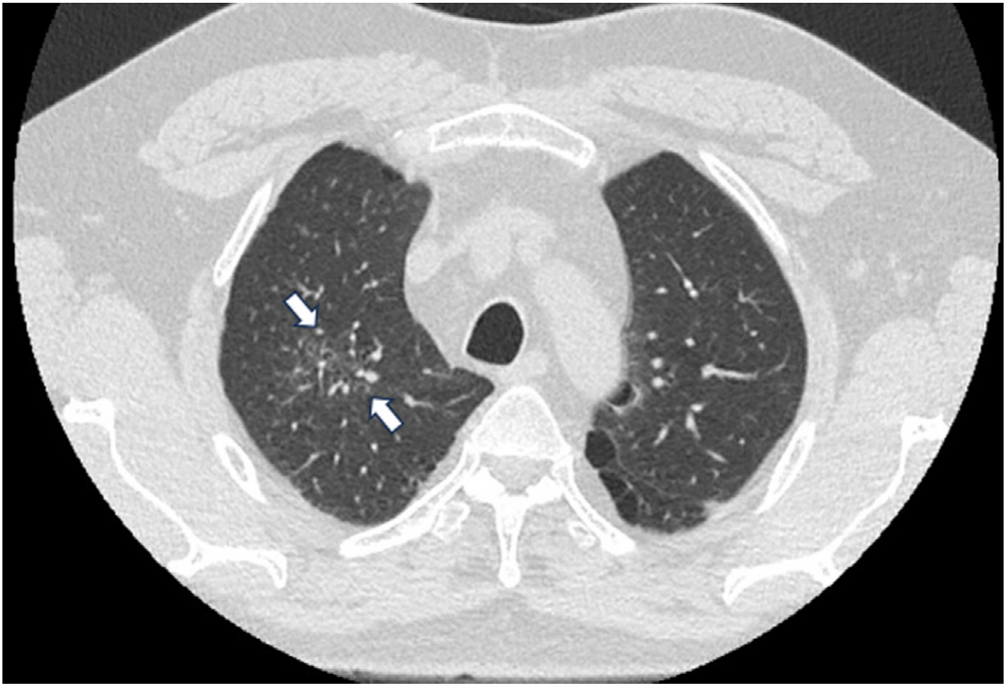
Chest computed tomography scan without contrast. Central right upper lobe ground glass opacity with associated reticulation and traction bronchiectasis (*indicated by white arrows*).
